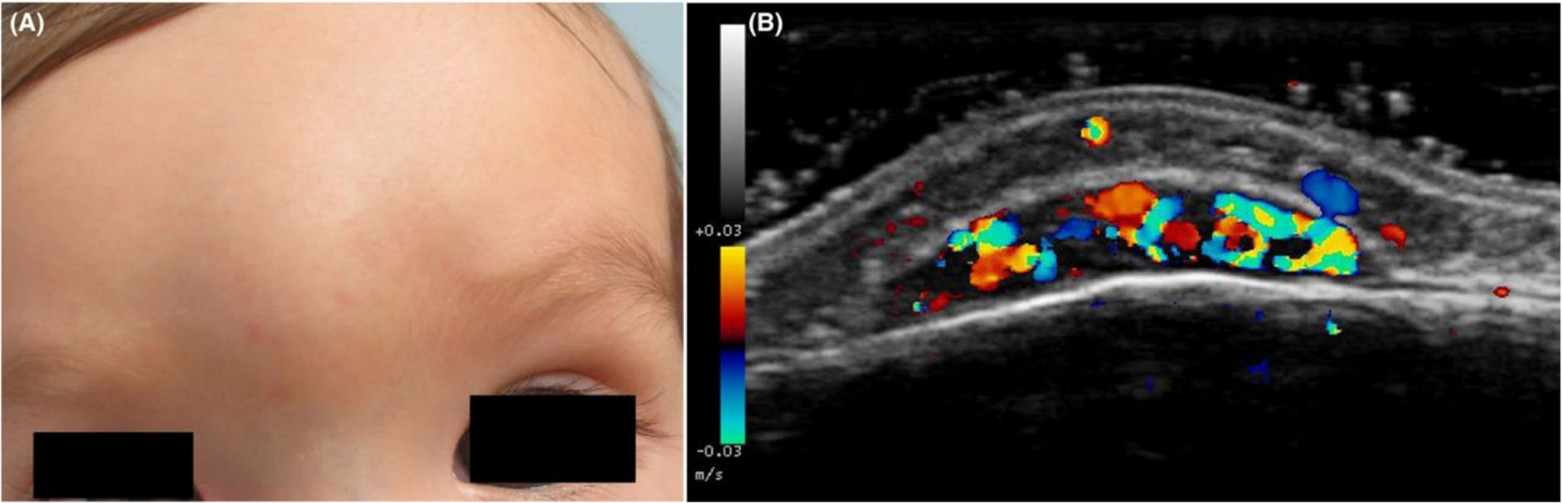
Infantile hemangioma. a Clinical picture shows a skin-colored soft mass with some subtle superficial telangiectasias on the glabella of a 7-month-old twin boy. b High-frequency ultrasonography (18 MHz) image (Doppler mode) reveals a subfascial hypoechoic well-defined hypervascular lesion with turbulent blood flow. The underlying frontal bone appears unaffected (Bandera, 2019). Reprinted with permission from Wiley Online Library [42]
